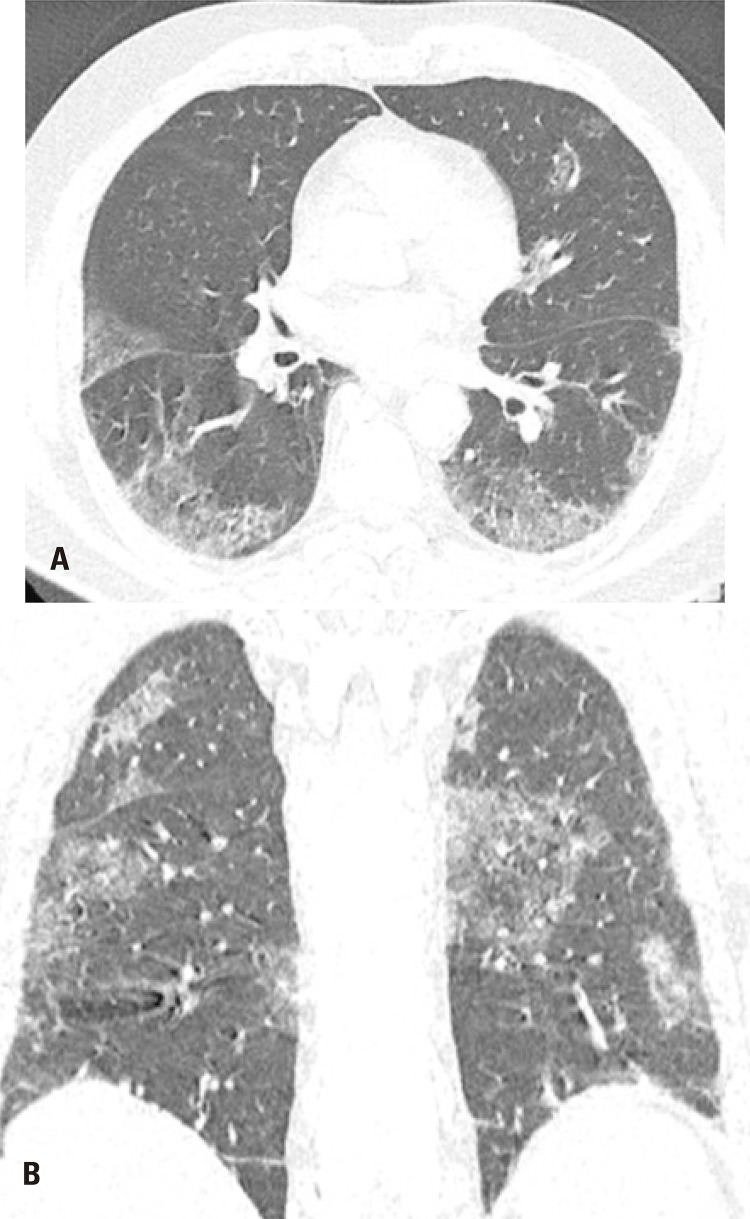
A 68-year-old male patient presented with dry cough, fever, myalgia and dyspnea. Computed tomography (A) Axial section and (B) Coronal reformation image: multiple ground-glass pulmonary opacities, sometimes associated with thickening of interlobular septa and intermingled reticulate, in multifocal, bilateral, predominantly peripheral and posterior distribution
